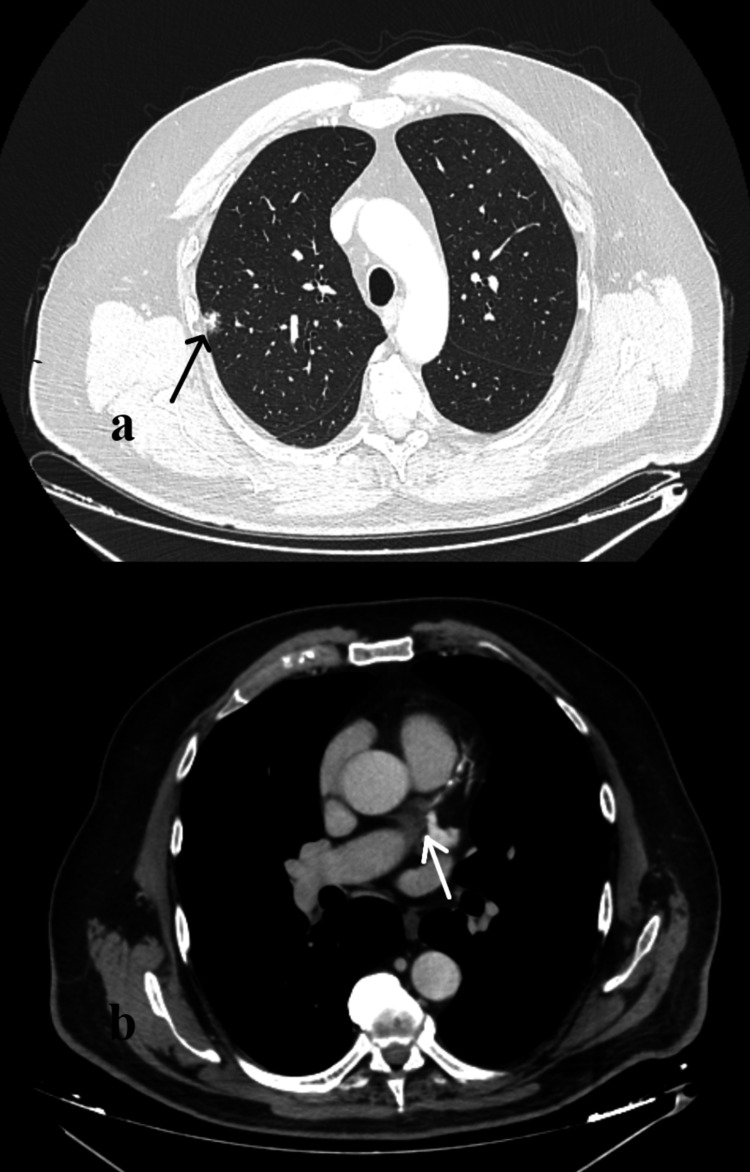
Chest CT (axial view) in the patient with T-PPL (a,b) (a) The black arrow shows a nodule with a halo sign. (b) The white arrow shows mediastinal lymphadenopathy.
